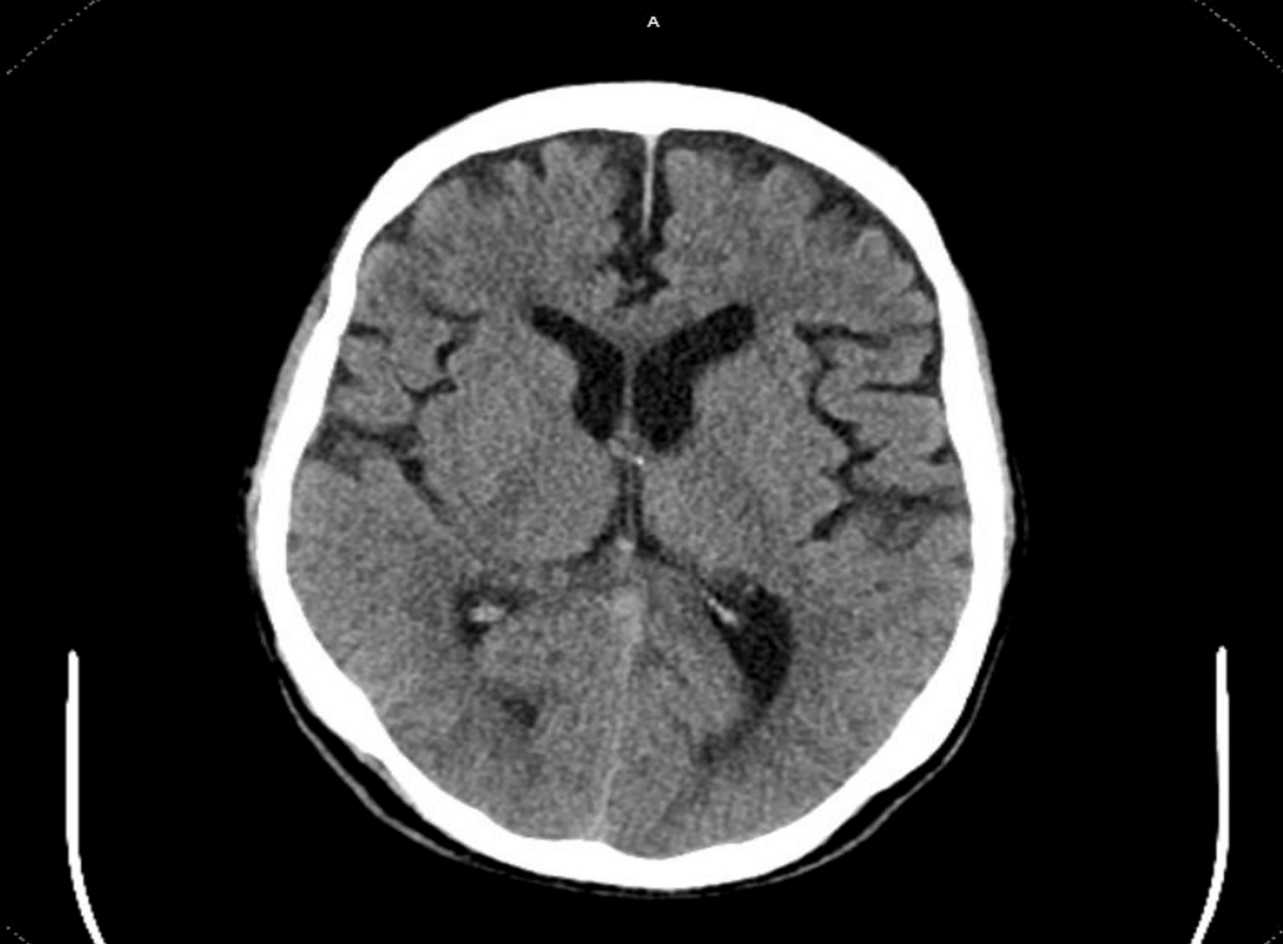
Computed tomography (CT) of the brain without intravenous contrast Axial brain scans reveal no signs of acute intracranial pathology. This includes a normal density of the brain parenchyma, the absence of acute intracranial hemorrhage, and no indications of abnormal masses, growths, mass effect, midline shift, or hydrocephalus.
